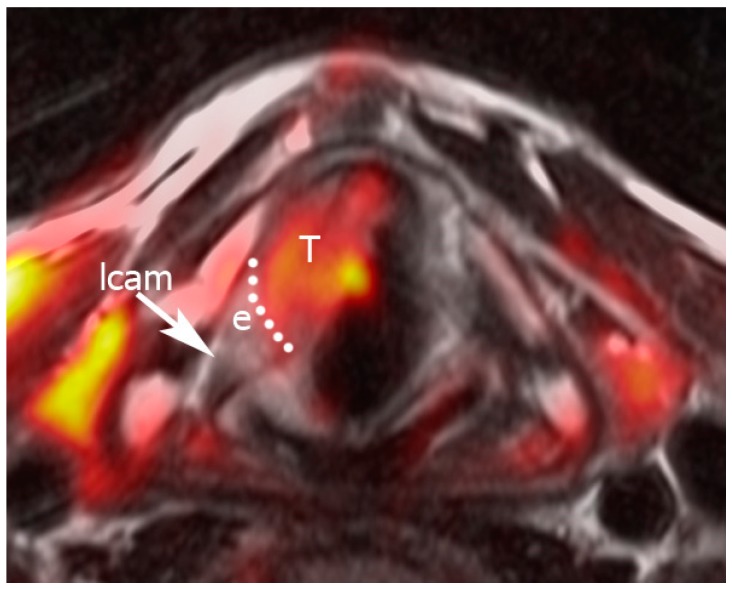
Example of Pattern B, indicating fusion between a T2-weighted sequence and a diffusion-weighted imaging (DWI) image. “Hot” areas correspond to the anterior glottic tumor. A dotted line separates the tumor from the peritumoral edema. The lateral cricoarytenoid muscle is normal. Legend: e, edema; lcam, lateral cricoarytenoid muscle; T, tumor.
